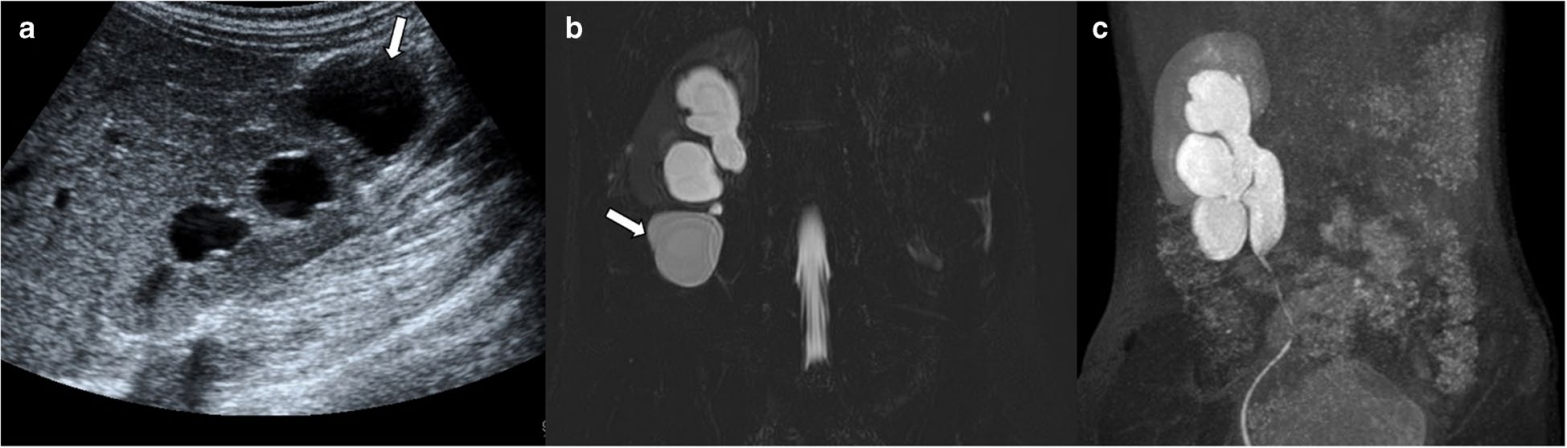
Crossed fused renal ectopia. An 8-year-old boy presented to the hospital with abdominal pain and ultrasound (US) was performed. a Longitudinal grayscale US image shows a thick-walled cystic lesion (*arrow*) in the inferior pole of the right kidney. The left kidney was not visualized in the left renal fossa. b Coronal T2-weighted maximum intensity projection (MIP) image demonstrates a cystic lesion (*arrow*) in the inferior pole of the right kidney that is not connected to the collecting system. c In the delayed phase, coronal post-contrast T1-weighted MIP image reveals contrast retention in the cystic lesion, indicating the dilated pelvicalyceal system of the ectopic left kidney
